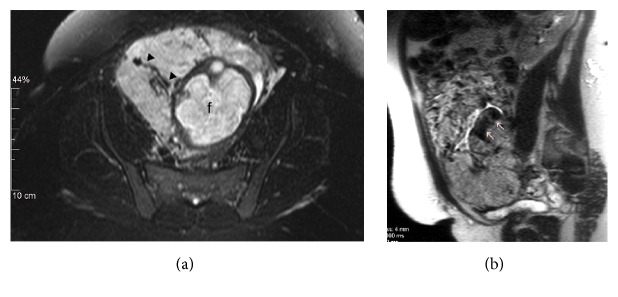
MRI of the pelvis without contrast performed 11 weeks later. (a) Transverse T2 fat suppressed image demonstrates enlarged intraplacental vessels (arrowheads) (f: fetal brain). (b) Sagittal T2 HASTE image shows thin uterine wall with dark intraplacental bands (arrows).
